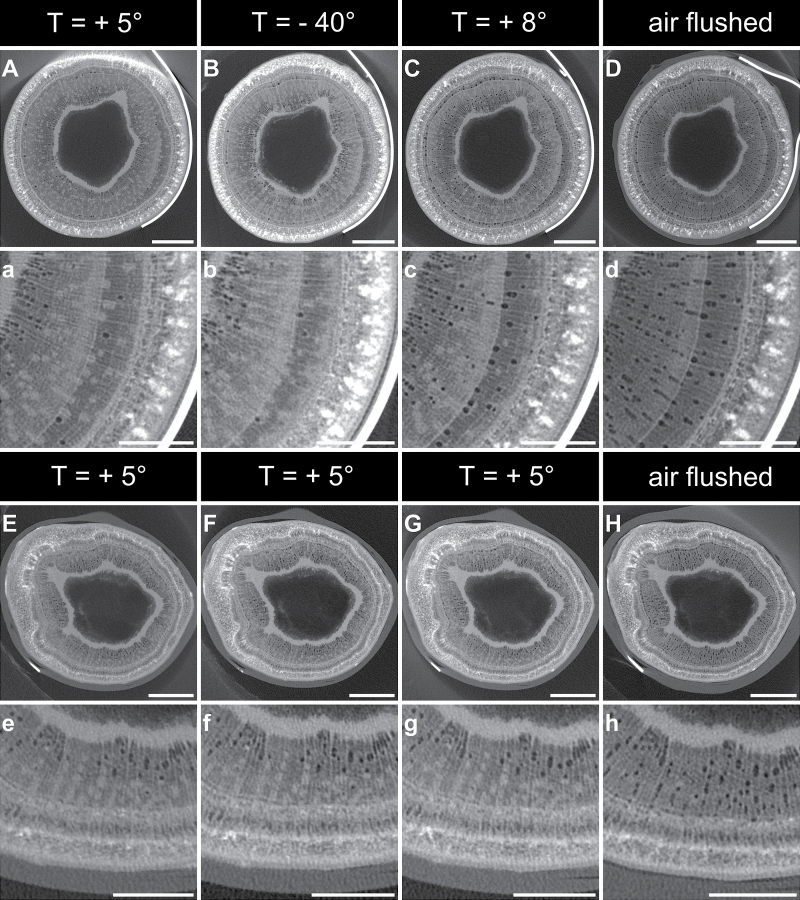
Transverse cross-sections of stem segments observed by X-ray microtomography during a freeze–thaw cycle, showing whole cross-sections (upper rows) and a more detailed view (lower rows). Scans were performed at the temperatures indicated during one freeze–thaw cycle for each sample (*n*=5; A–C, a–c) and control samples (*n*=3; E–G, e–g) were scanned at the same times (*t*=0, 18, and 36h) but were kept at a constant temperature (+5 °C). D and H represent respectively the Scans of air-flushed treated samples and control samples are shown in (D/d) and (H/h), respectively. Bars, 1mm.
